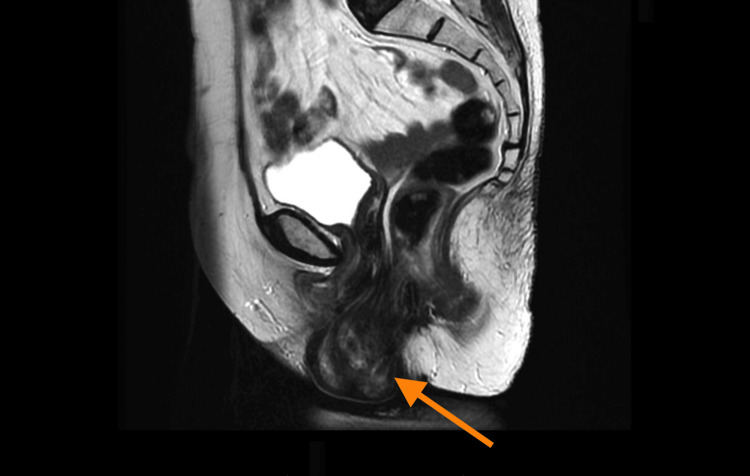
Preoperative MRI showing rectovaginal prolapse with herniation of abdominal contents into the pelvic cavity
